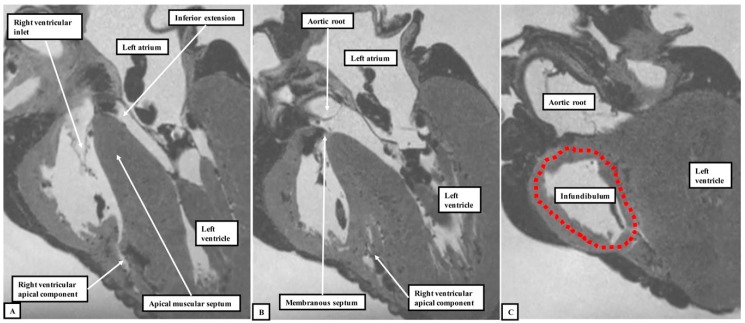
Panels A through C show the images obtained by scanning the heart photographed in Figure 1 and Figure 3 using magnetic resonance imaging prior to subsequent dissection. They show that, by virtue of the inferior extension of the subaortic outflow tract, the apical muscular ventricular septum separates the inlet of the right ventricle from the outlet of the left ventricle. They also reveal that the subpulmonary infundibulum is a myocardial sleeve, as shown by the dotted red oval in panel C.
